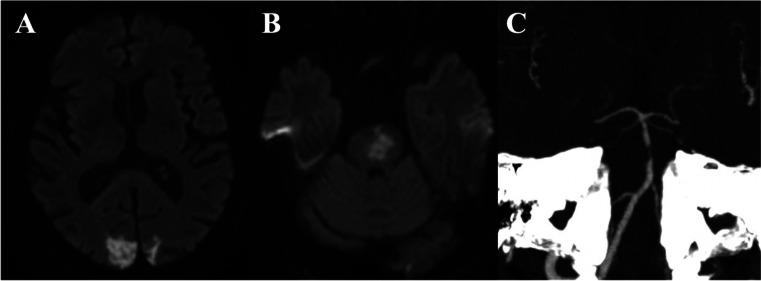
Brain magnetic resonance imaging showed recent ischemic lesions in the occipital lobes bilaterally (panel A, diffusion-weighted sequences) and in the pons (panel B, diffusion-weighted sequences). Head computed tomography angiography showed a stenosis of the middle third of the basilar artery (panel C)
